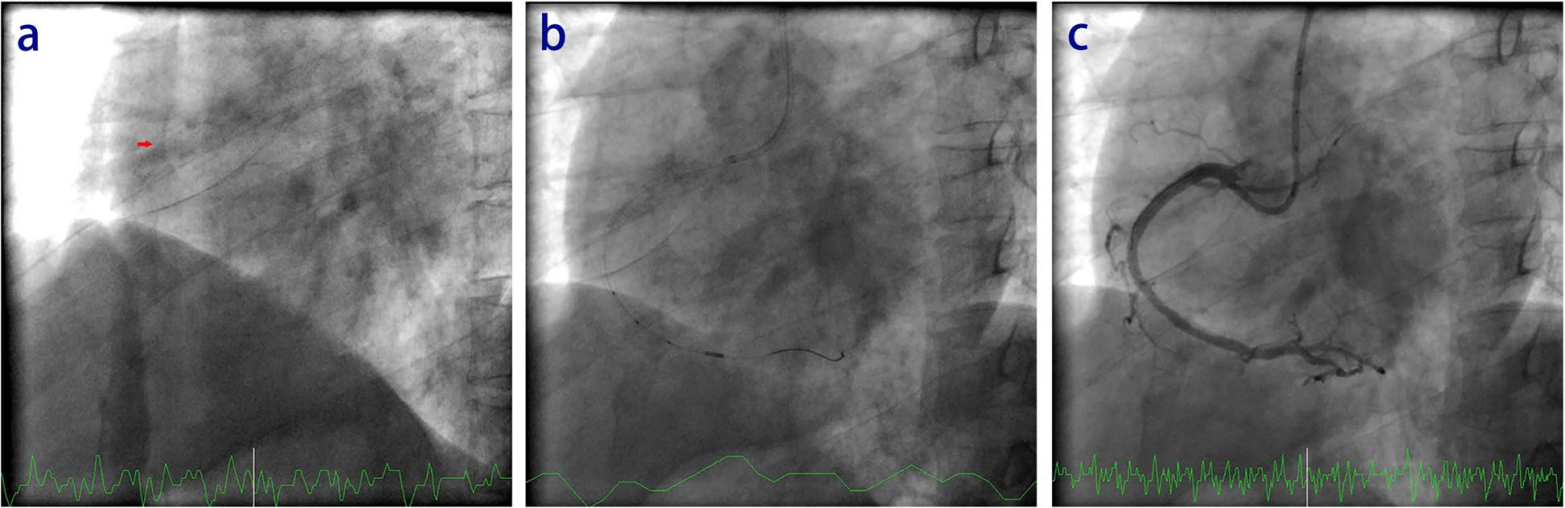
Post-PCI angiography of the RCA with IVUS detection. a Radiography of the RCA was performed to confirm that the stent was still inside the proximal RCA. The red arrow points to the stent. b Intravascular ultrasound was performed to check the stents in the RCA. c Angiography was finally performed to check the RCA
